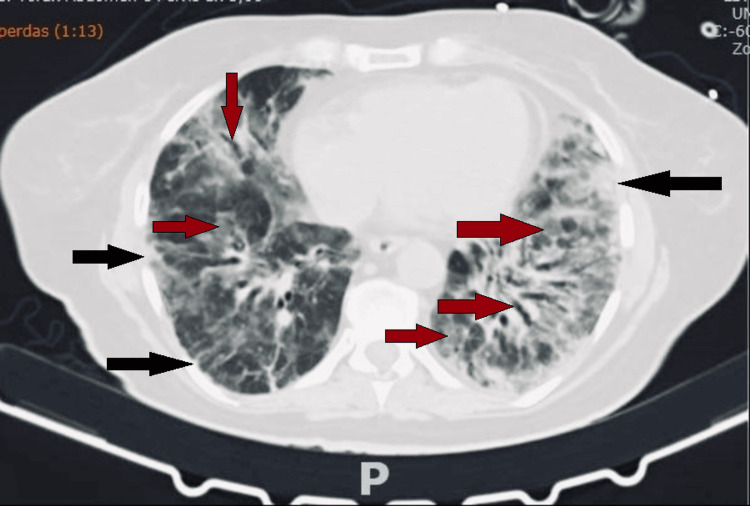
Third lung CT scan (axial view) CT: Computed Tomography The black arrows show subpleural consolidations in the right and left lung; the red arrows show ground-glass opacities and bronchiectasis in the right and left lung.
